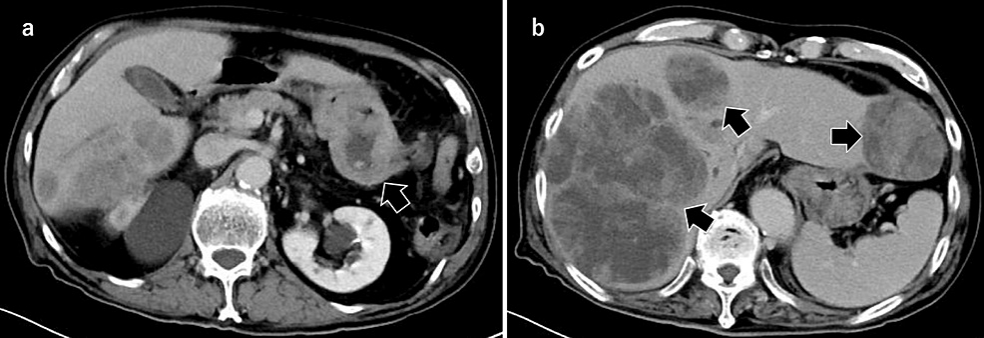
(a) Irregular wall thickening and increased adipose tissue concentration were observed from the gastric body to the angular notch (arrow). (b) Contrast-enhanced computed tomography (CT) revealed multiple tumors (arrows). All tumors appeared as low-density areas on contrast-enhanced CT.
